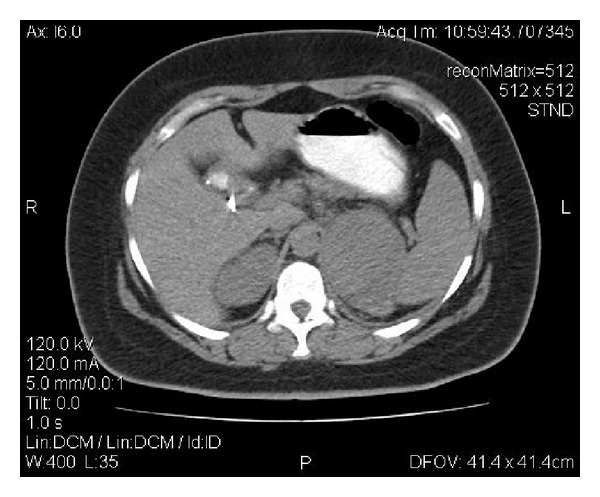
A CT scan image showing the adrenal tumor.
